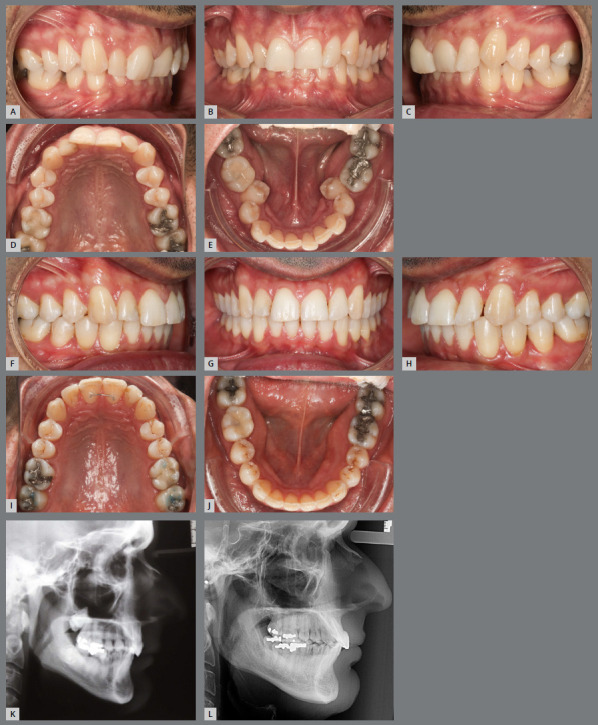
Initial images of a patient with Angle Class II, division 2 malocclusion, increased overbite, with lingually inclined upper and lower incisors (A-E); and after orthodontic treatment (F-J), with corrected axial inclinations of the posterior teeth. The initial and final lateral cephalometric radiographs (K,L), demonstrate the correction of the axial inclinations of upper and lower incisors.
